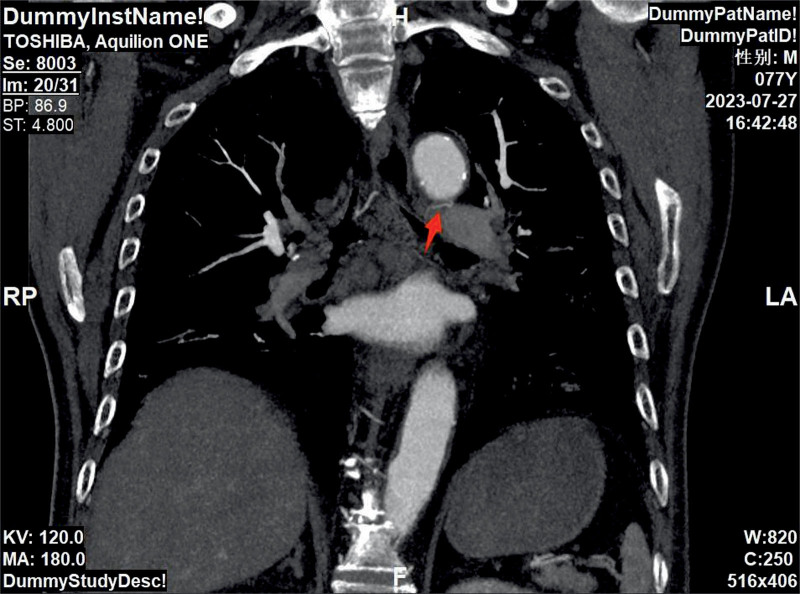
Computed tomography angiography (CTA) of the pulmonary artery showed thickening of the left bronchial artery, with the abnormal vessel marked by an arrow.
